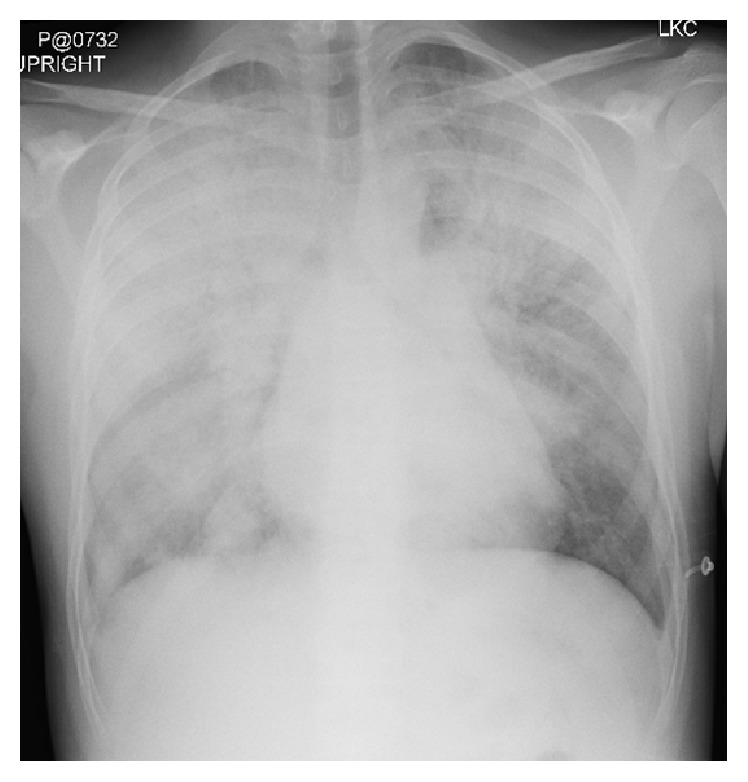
Chest X-ray at presentation demonstrating extensive airspace disease that was interpreted as multifocal pneumonia versus pulmonary edema.
